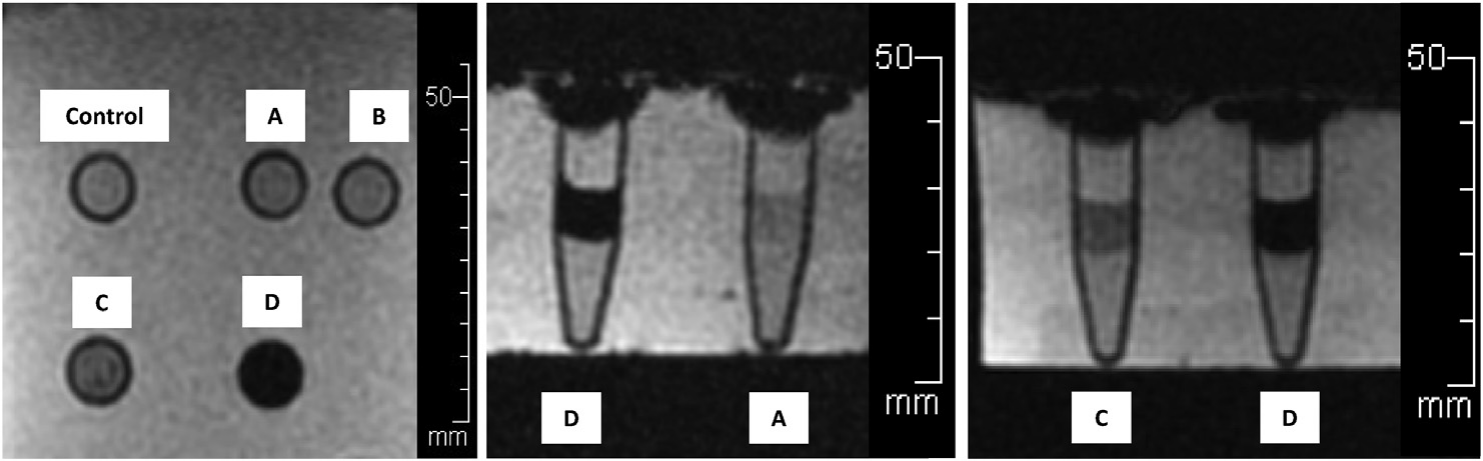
IS-labeled ASCs from T2∗-weighted sequences of 1.5T MRI. Left: Axial view, Middle: Sagittal view, Right: Coronal view. (A) 1 × 10^3^ cells/ml/microtube, (B) 1 × 10^4^ cells/ml/microtube, (C) 1 × 10^5^ cells/ml/microtube, (D) 1 × 10^6^ cells/ml/microtube.
